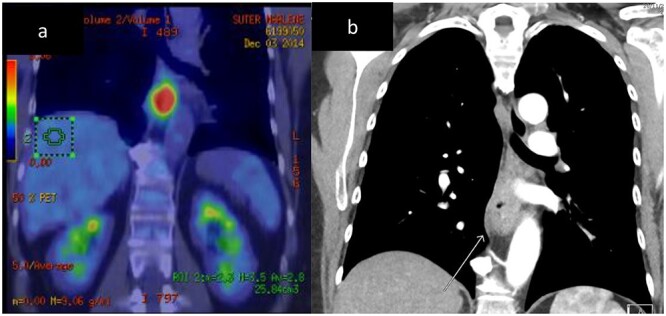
Case 1. CT and FDG-PET imaging of primary oesophageal tumour. Thickening of the oesophageal wall (a) and intense uptake on FDG-PET (b).
